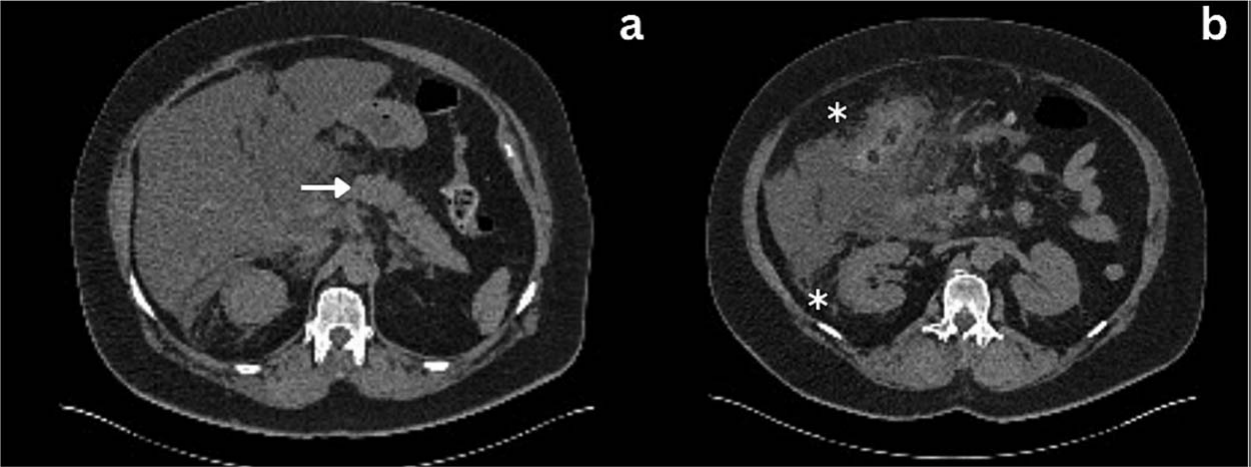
Axial section CT imaging; a) Normal pancreas with no evidence of inflammation or malignancy (arrow), b) extrahepatic biliary collection (asterisks).
